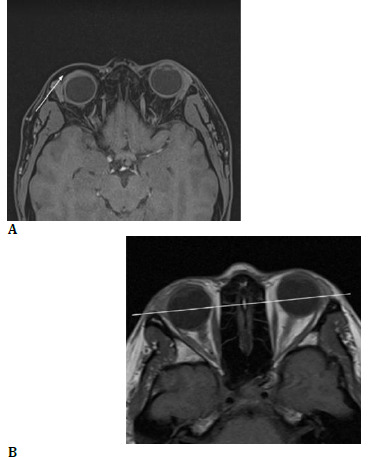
A, B Orbital MRI showing eyelid fat infiltration, intraconal fat loss, and enophthalmos
